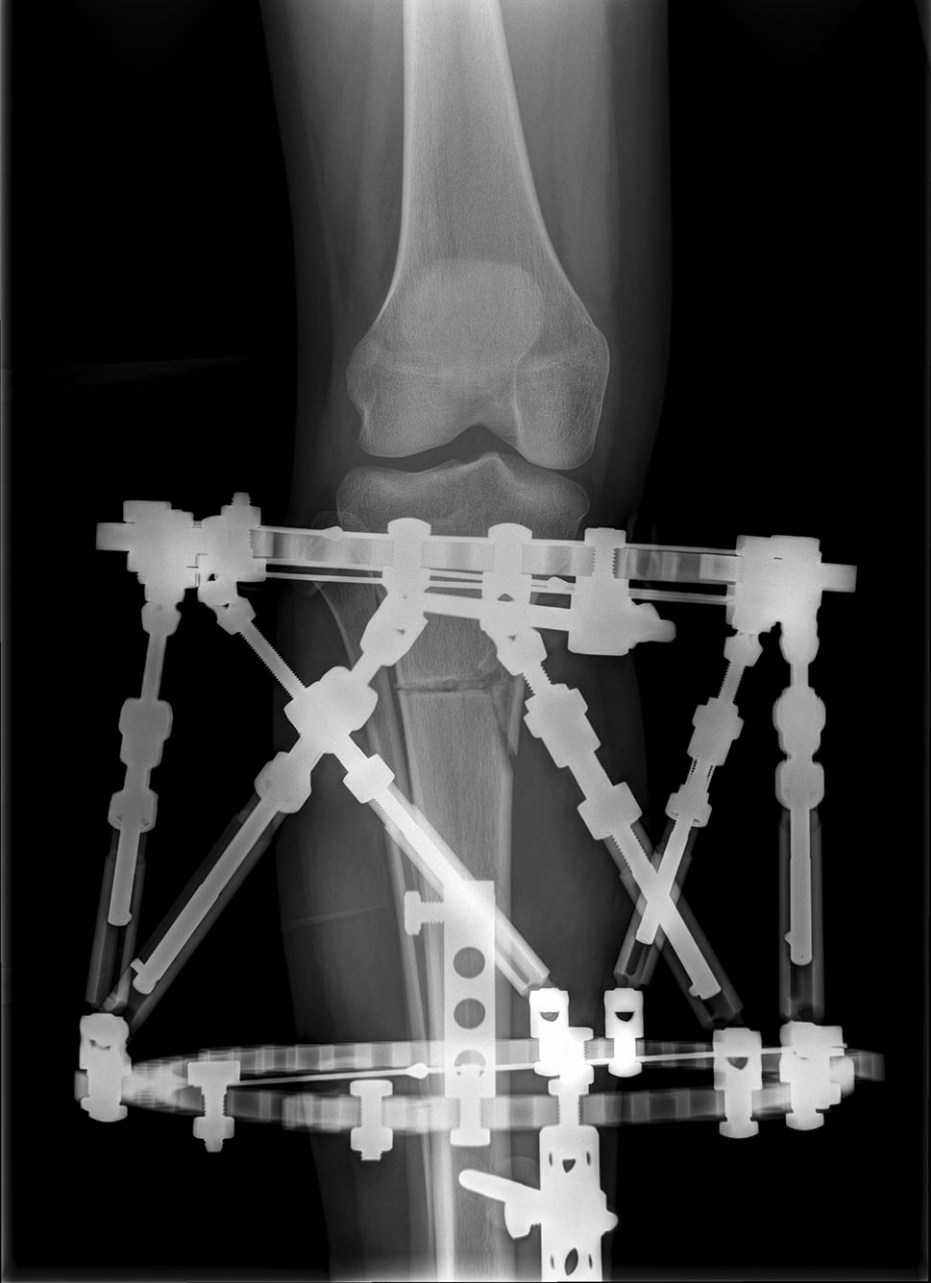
AP radiograph of the right tibia: an adequate radiograph taken orthogonal to and including the entire reference ring allowed sufficient visualisation of any deformity and the osteotomy
